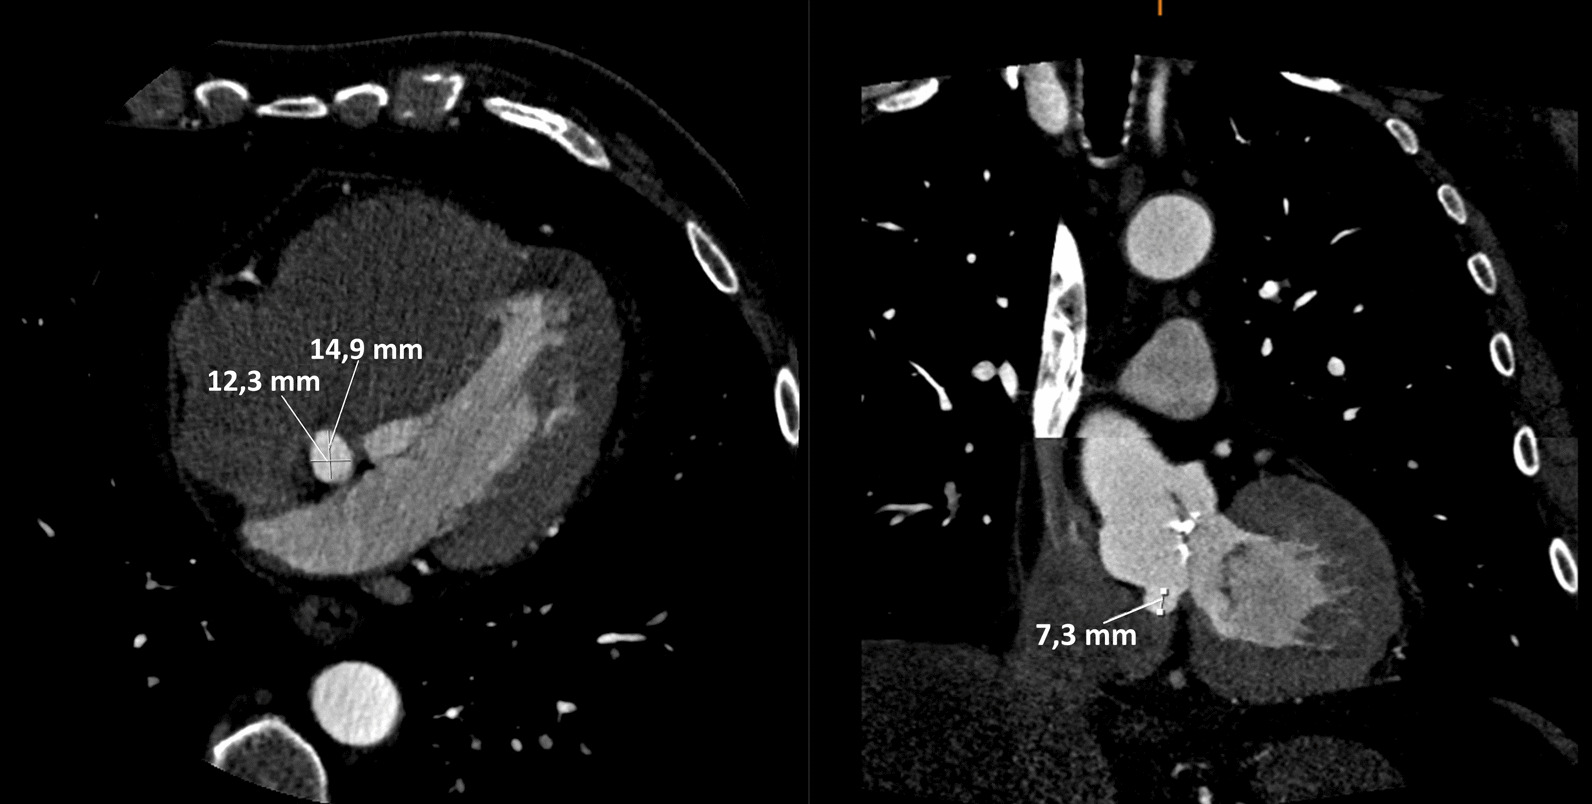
CT-scan measurements of the false aneurysm (contained aortic rupture)
